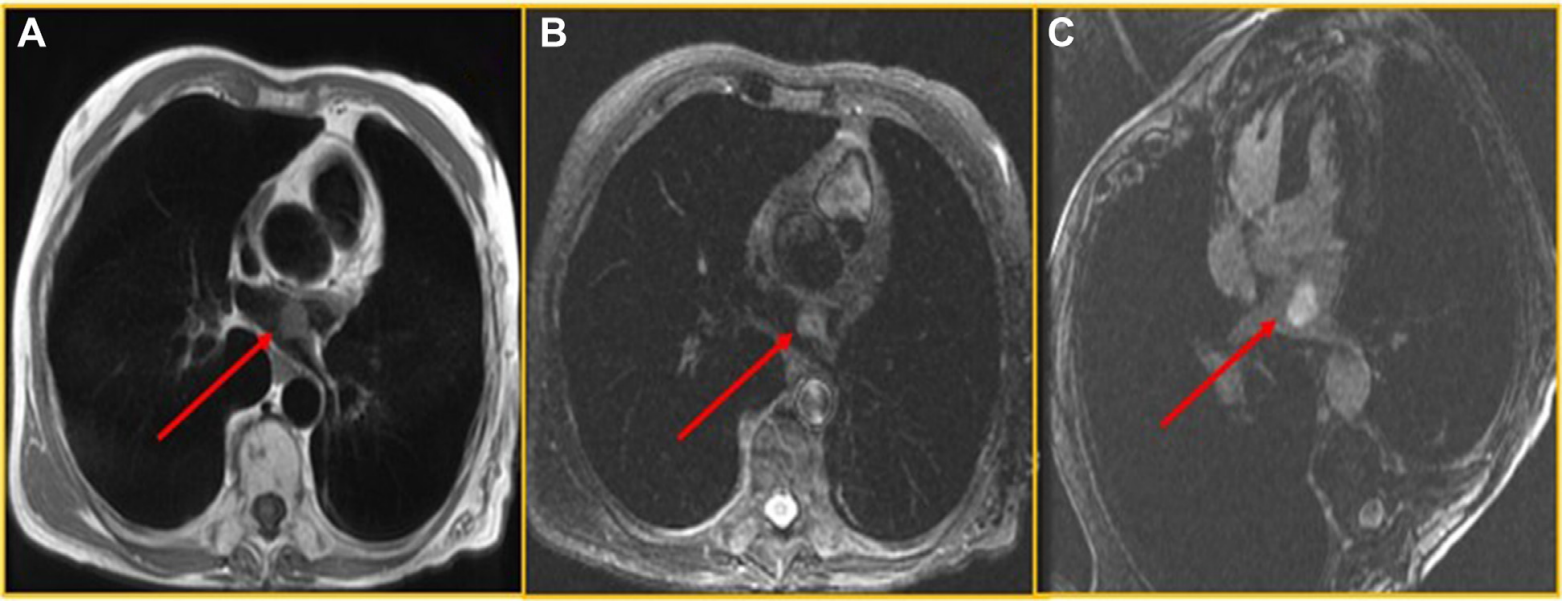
Cardiac Magnetic Resonance (A) T1-weighted image showing iso-intense mass in the LA lateral wall. (B) T2-weighted image showing mildly hyperintense at the lateral wall of the LA. (C) Delayed myocardium enhancement imaging shows avidly enhancing mass in the LA. Abbreviations as in Figure 1.
